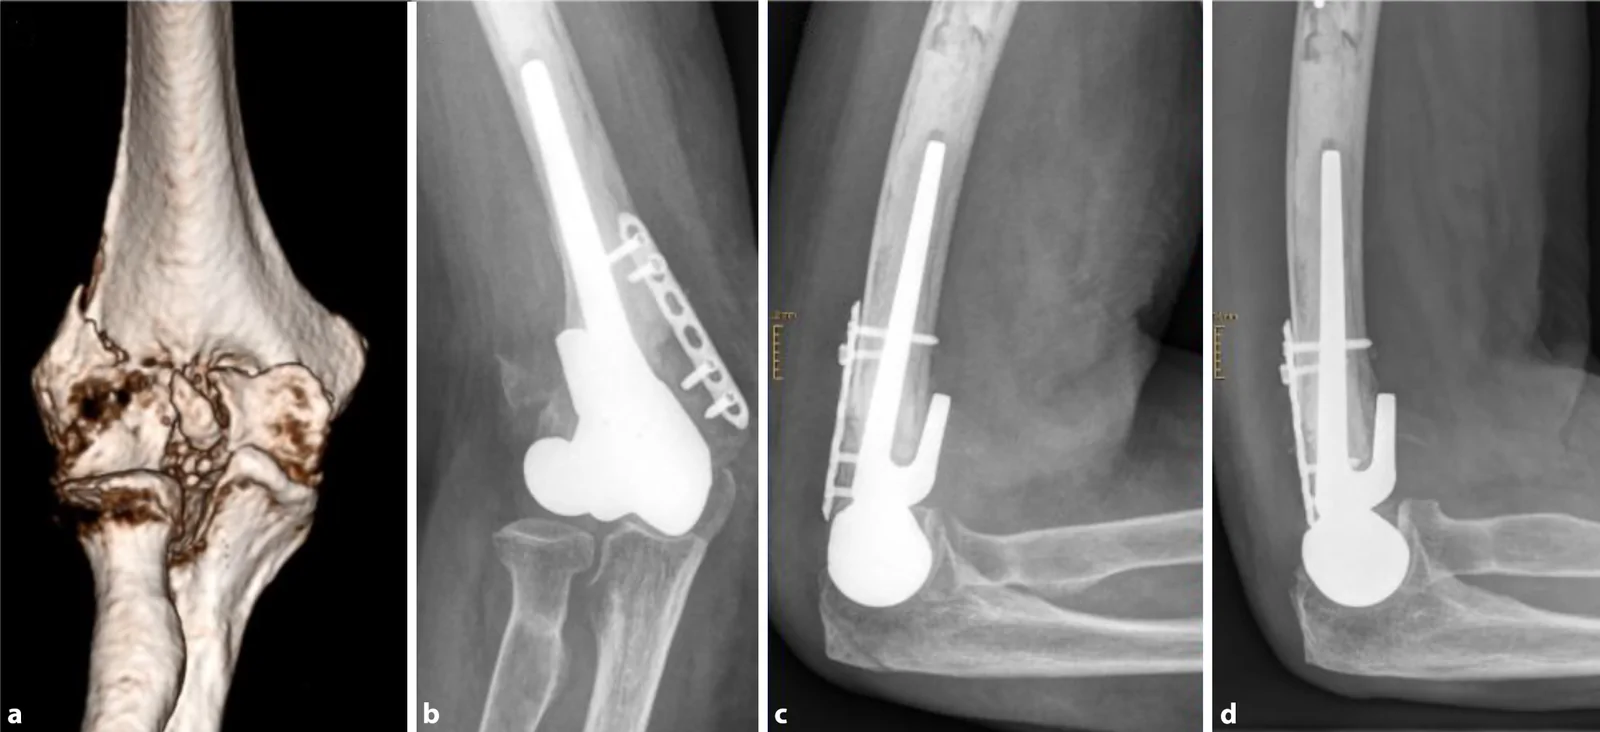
A 77-year-old woman sustained a distal intra-articular humerus fracture which was treated with a hemi-prosthesis and additional plate osteosynthesis. a Preoperative computed tomography. b, c X-rays obtained at the 3‑month follow-up. The flip osteotomy is still visible. d X-ray from the 6‑month follow-up showing the healed flip osteotomy
